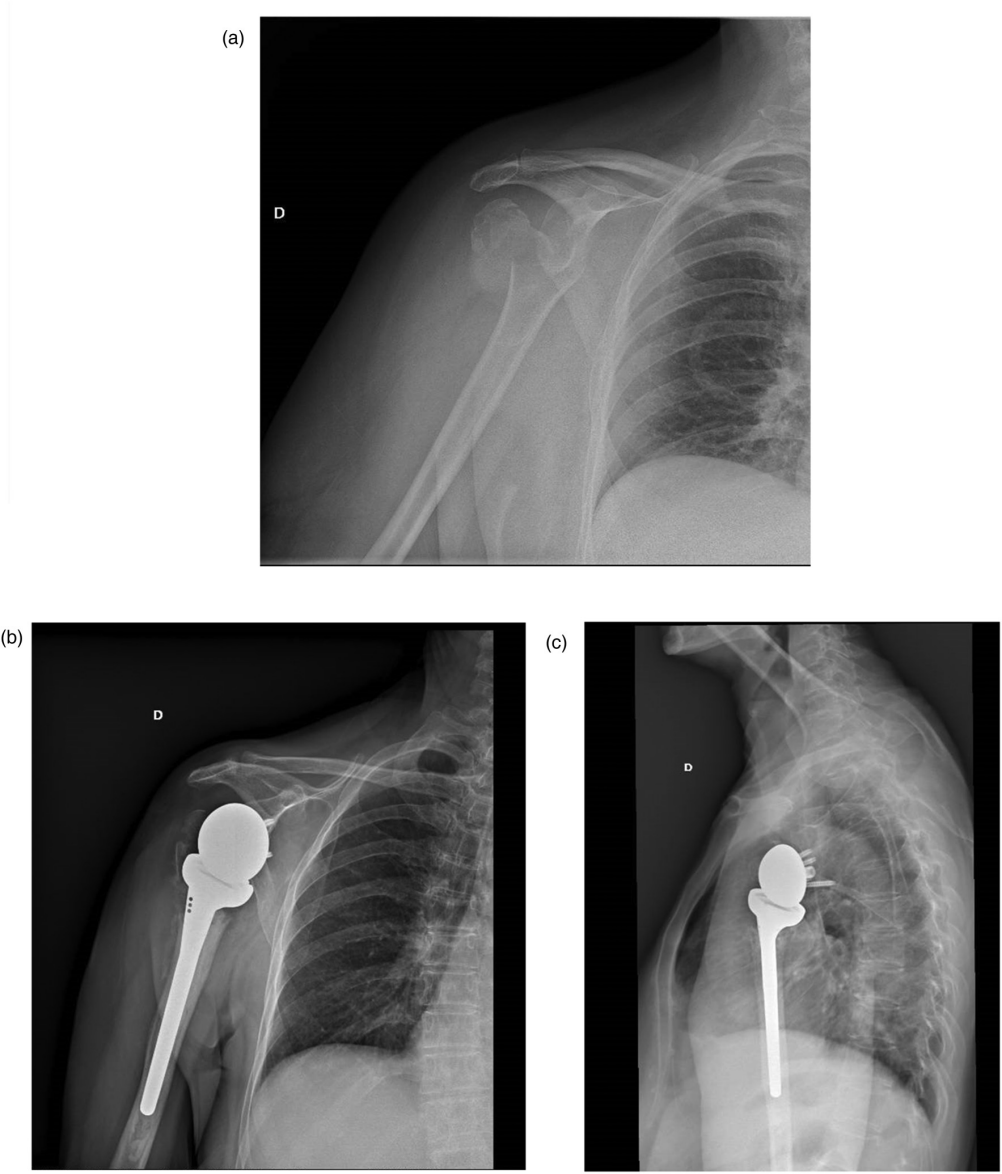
81-year-old female who suffered a 4-part proximal humerus fracture, anteroposterior radiograph same day of injury (A), anteroposterior (B), and transthoracic (C) views during follow-up.
